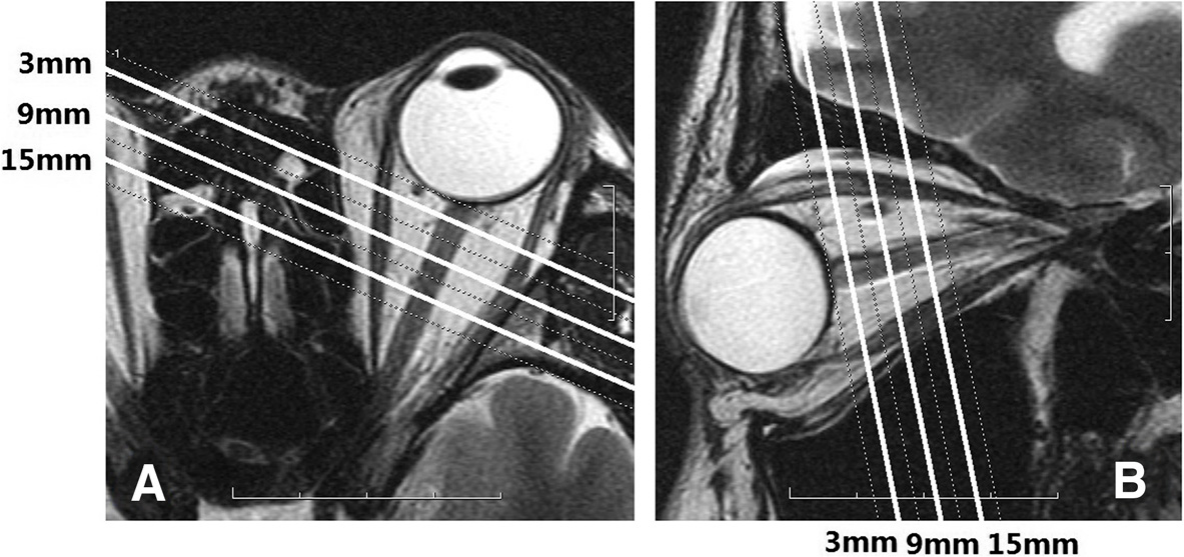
Magnetic resonance imaging scan of the retrobulbar optic nerve ((A) transversal section, and (B) oblique sagittal section) T2WI-FRFSE of the retrobulbar optic nerve (digital field of view = 8, window width = 2,000, window level = 1,000). These images were used to plan the position of three slices at 3, 9, and 15 mm behind the globe of T2WI-FRFSE fat-suppressed sequences to measure the optic nerve diameter, optic nerve sheath diameter, and width of the orbital subarachnoid space at 3, 9, and 15 mm behind the globe.
